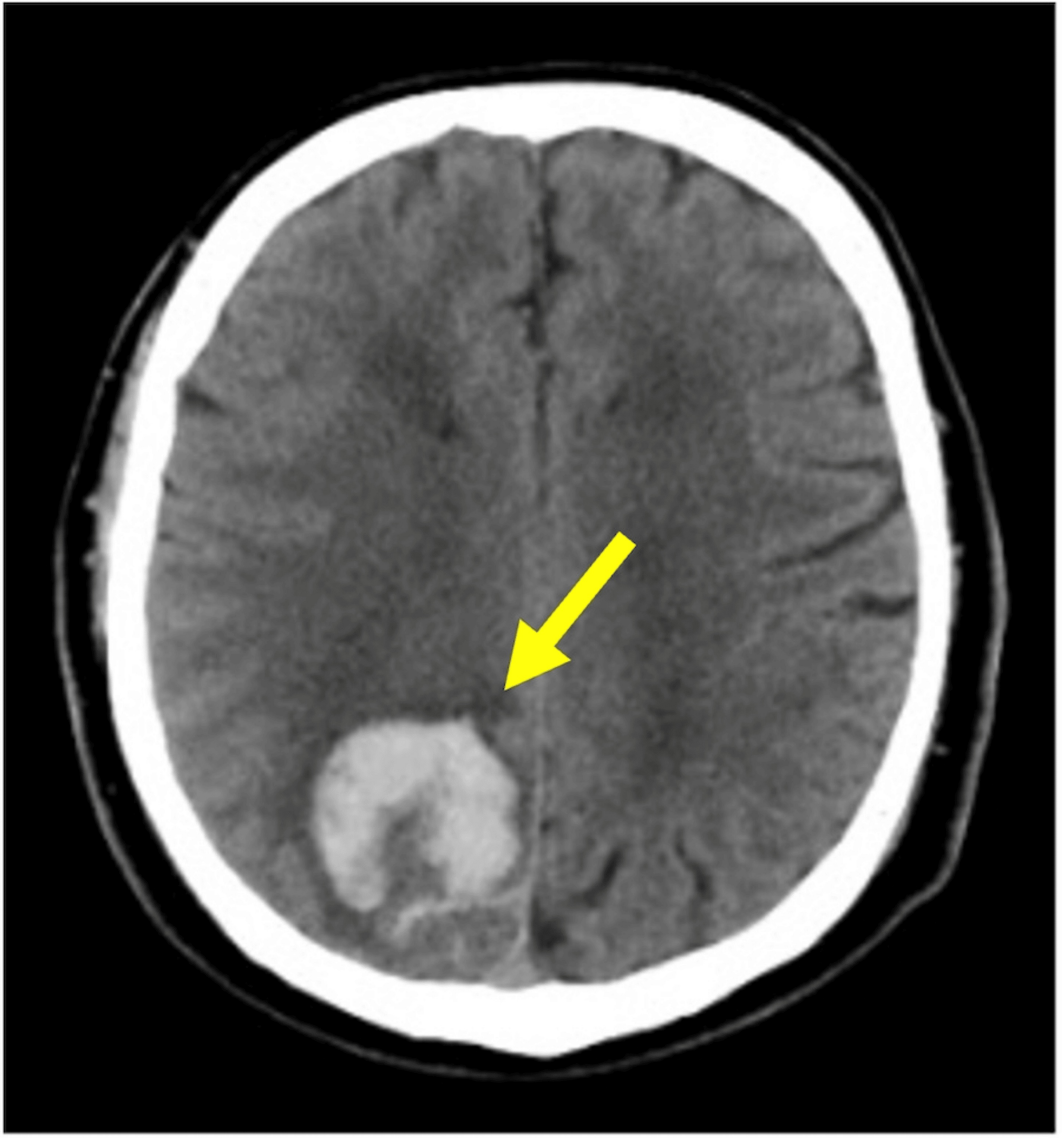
Brain image of the study patient. Computed tomography (CT) scan on day one post-stroke. The yellow arrow indicates the acute subcortical hemorrhage in the right superior parietal lobe.
